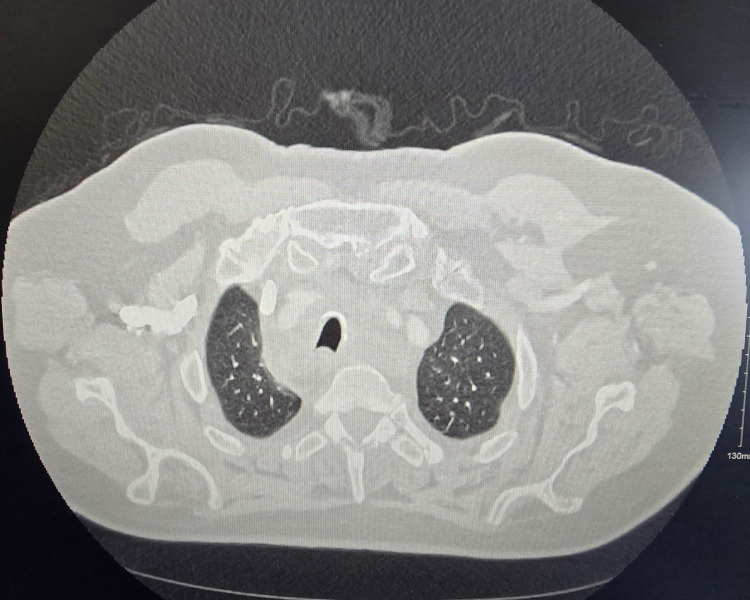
CT thorax with contrast showing slice at the level of the trachea
